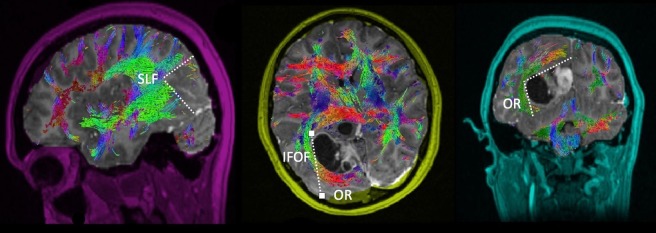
Anterior Occipital Diffusion tractography enhanced T1 magnetic resonance imaging (MRI) with contrast with (A) sagittal, (B) axial, and (C) coronal views demonstrating an anterior occipital glioma in relation to the surrounding white matter tracts. Labeled white matter tracts associated are shown as follows: inferior fronto-occipital tract (IFOF), superior longitudinal fasciculus (SLF), and optic radiations (OR). The disconnection pattern is shown as a white dotted line.
